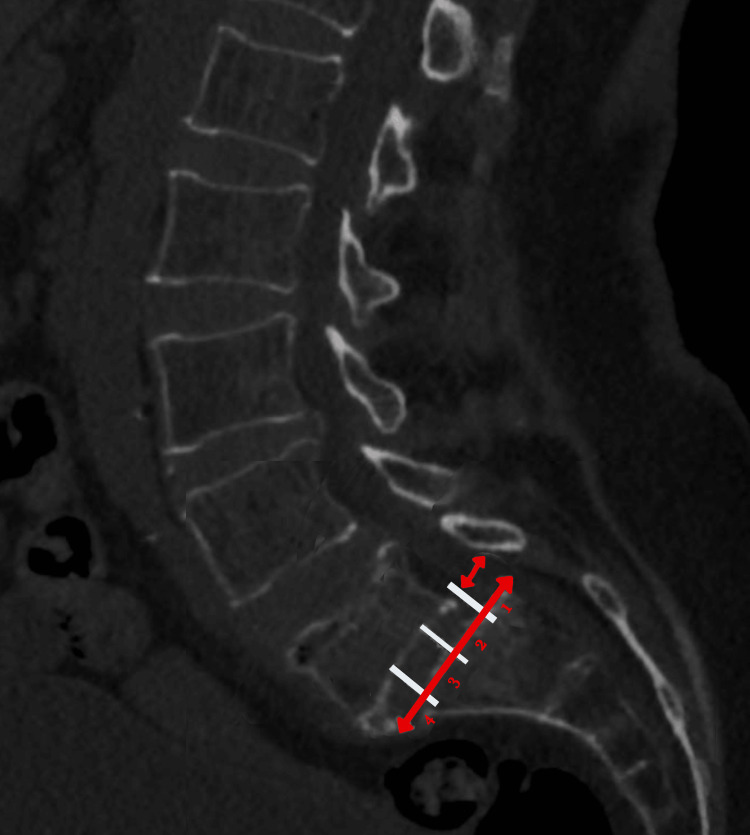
CT anterolisthesis grade I at the level of L5-S1
